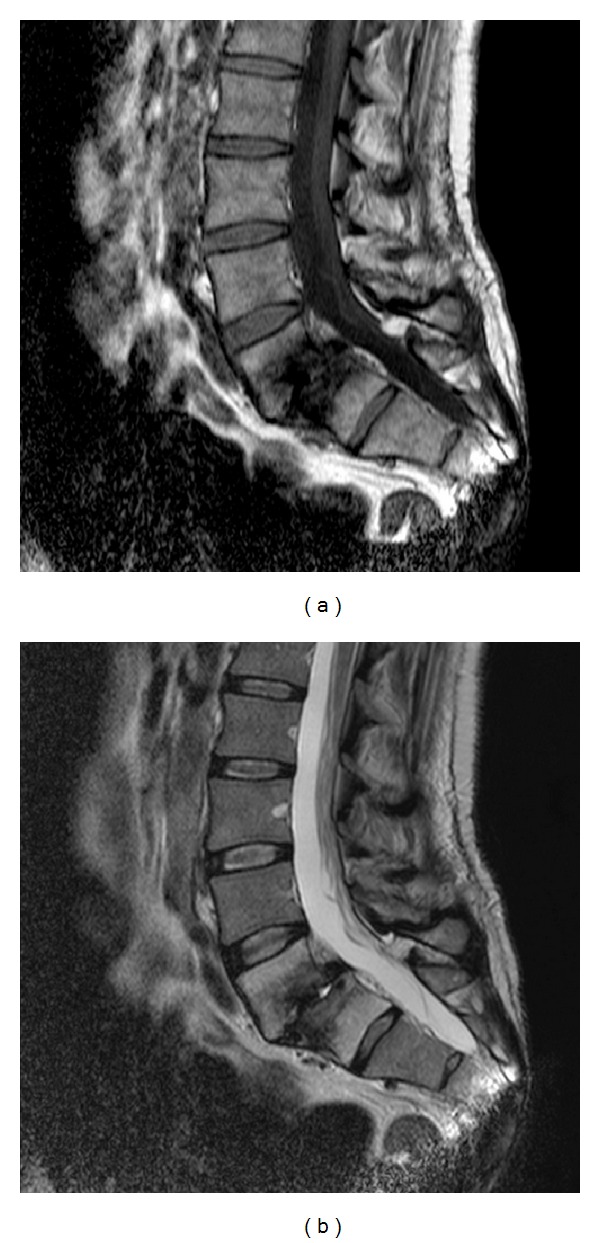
T1 (a) and T2 (b) sagittal lumbar MRI showing an L5/S1 severe discopathy with meyerding grade I listhesis and Modic type 3 sign.
